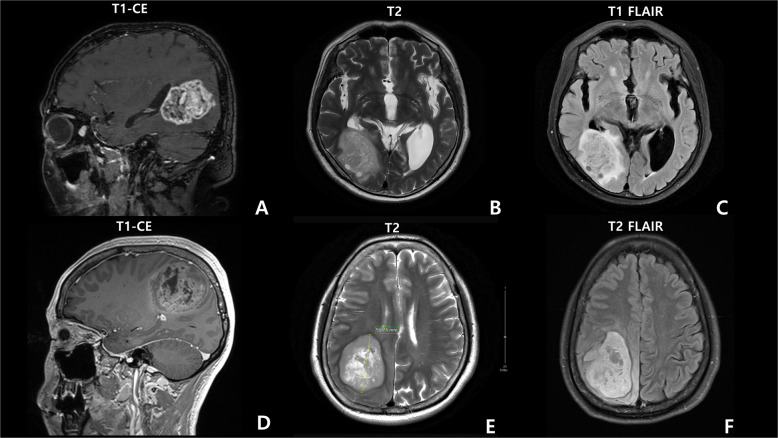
The brain MRI images of case 4 with Lynch syndrome. A sagittal T1-weighted (postcontrast), B axial T2-weighted, and C T2 FLAIR MRI results, showing an ~6 cm-long diameter enhancing mass with perilesional edema in the right occipital lobe. Case 2 (glioblastoma *IDH*-wildtype) D sagittal T1-weighted (postcontrast), E axial T2-weighted, and F T2 FLAIR MRI results, revealing an ~5.7 cm heterogeneous mass in the right parietal lobe and midline shift.
